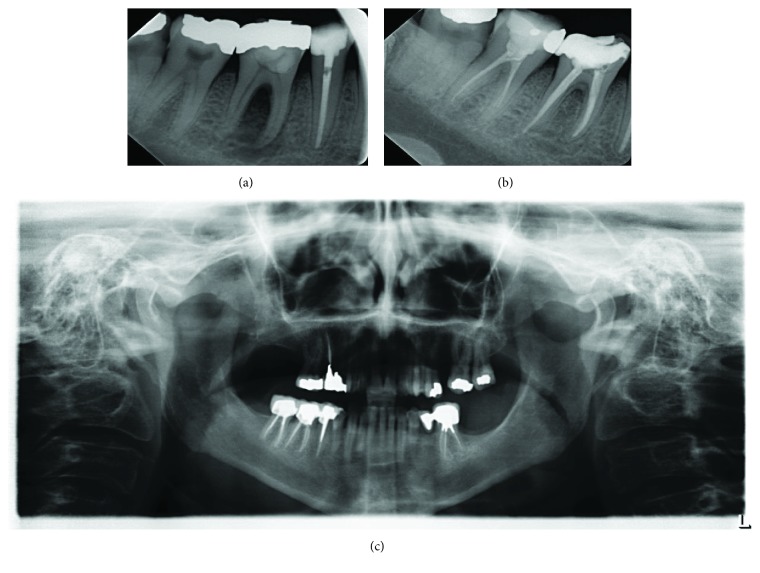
Case 1. (a) The initial radiograph of tooth #46 shows periapical and furcation bone resorption. (b) The 1-year recall radiograph shows healing of the bone lesion. (c) The 6-year follow-up radiograph shows tooth #46 with a permanent crown.
